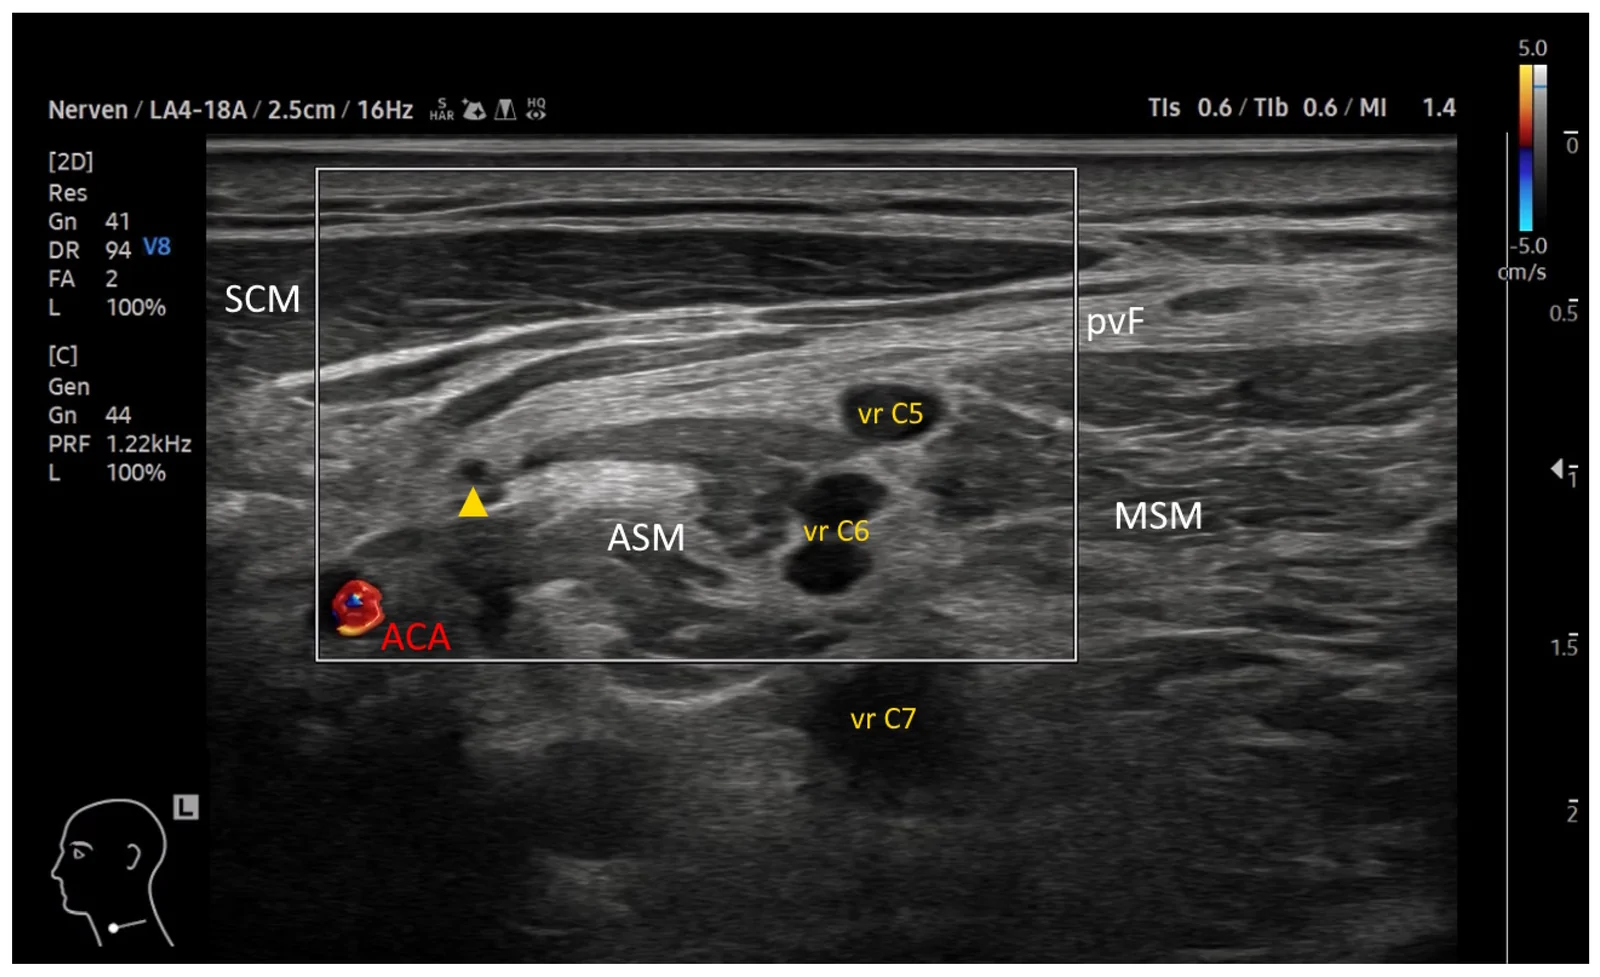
Interscalene level and proximity of the phrenic nerve. Ultrasound image obtained at the classical interscalene level demonstrating the ventral rami (vr) of C5–C7 located between the anterior scalene muscle (ASM) and middle scalene muscle (MSM). The phrenic nerve (yellow arrowhead) is visible beneath the prevertebral fascia (pvF) in close proximity to the brachial plexus within the same compartment. SCM = sternocleidomastoid muscle; ACA = ascending cervical artery.
